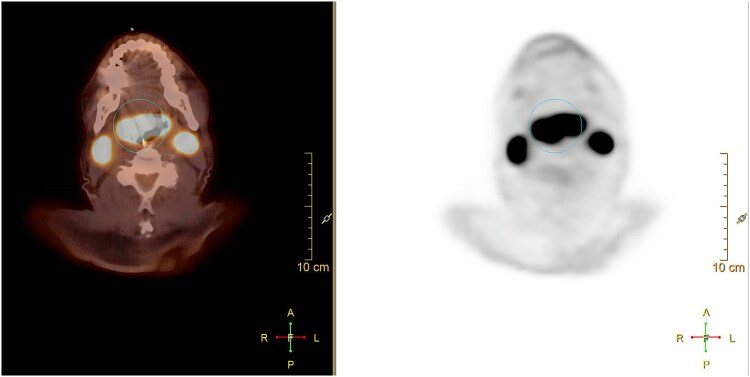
PET/CT showing hypermetabolic base of tongue mass with FDG avid bilateral level IIa cervical lymph nodes.
